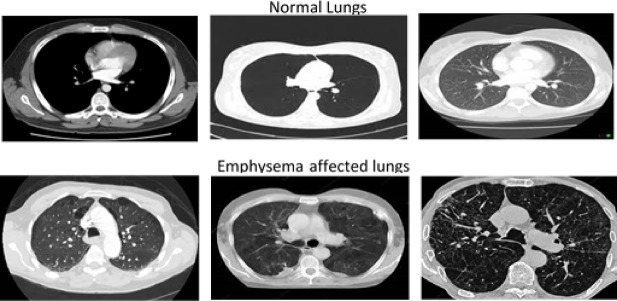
Sample CT scan slices of a normal lung and emphysema-affected lungs.
